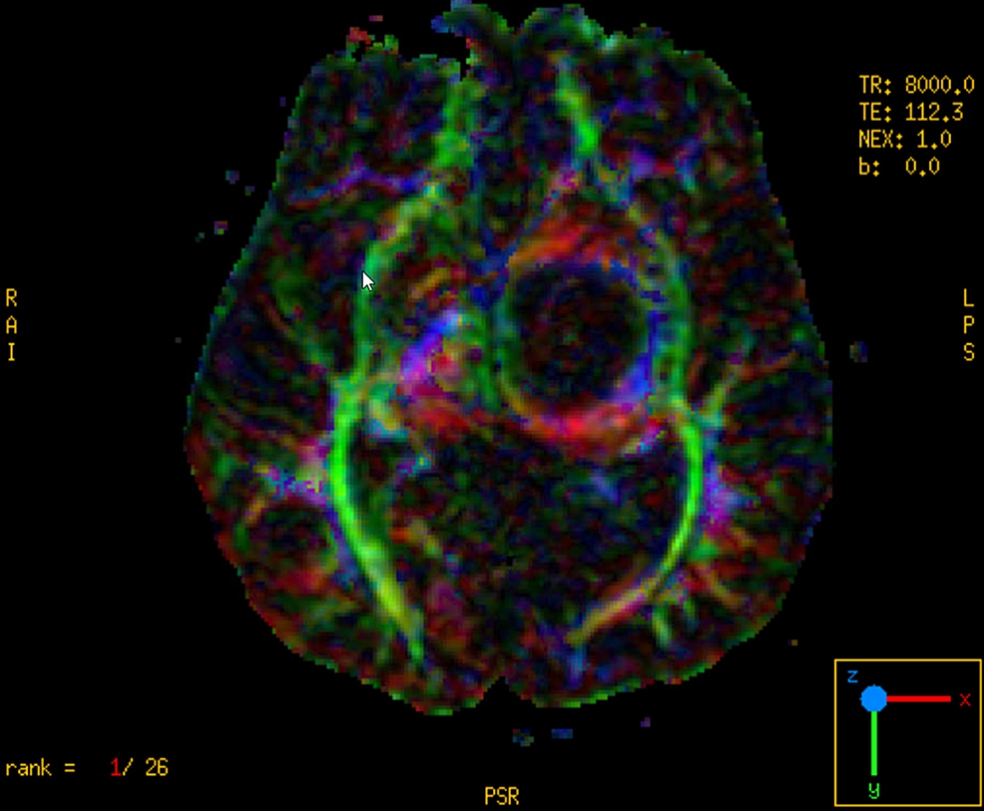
Preoperative axial MRI and DTI image shows deviation of PLIC posterolaterally. DTI: diffusion tensor imaging; PLIC:
